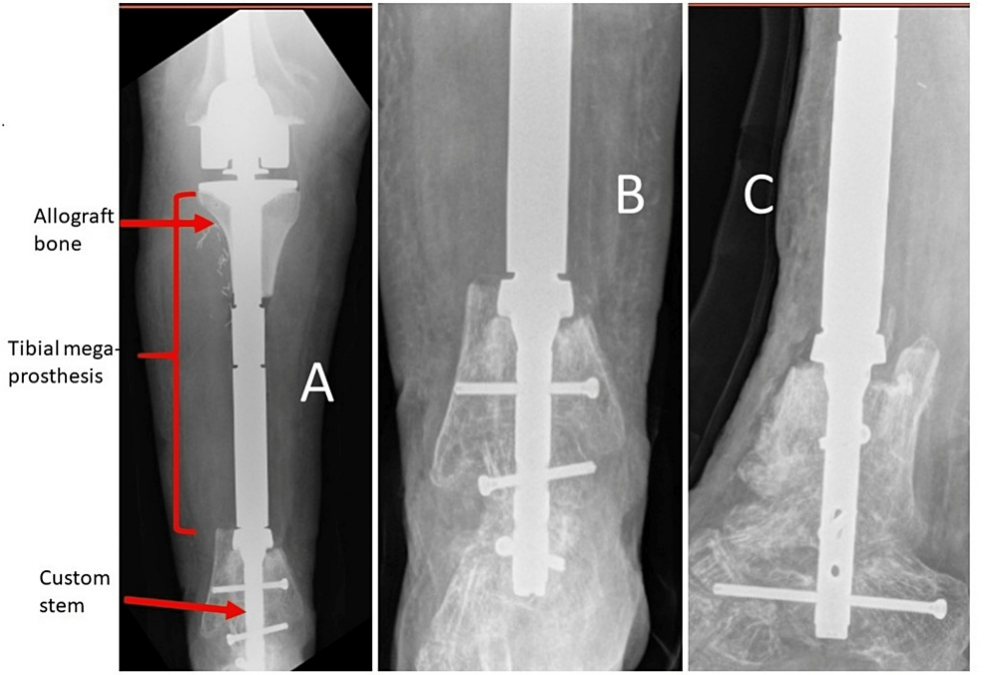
Radiographs of prosthetic (A) and the stem (B, C) for the tibial megaprosthesis.
